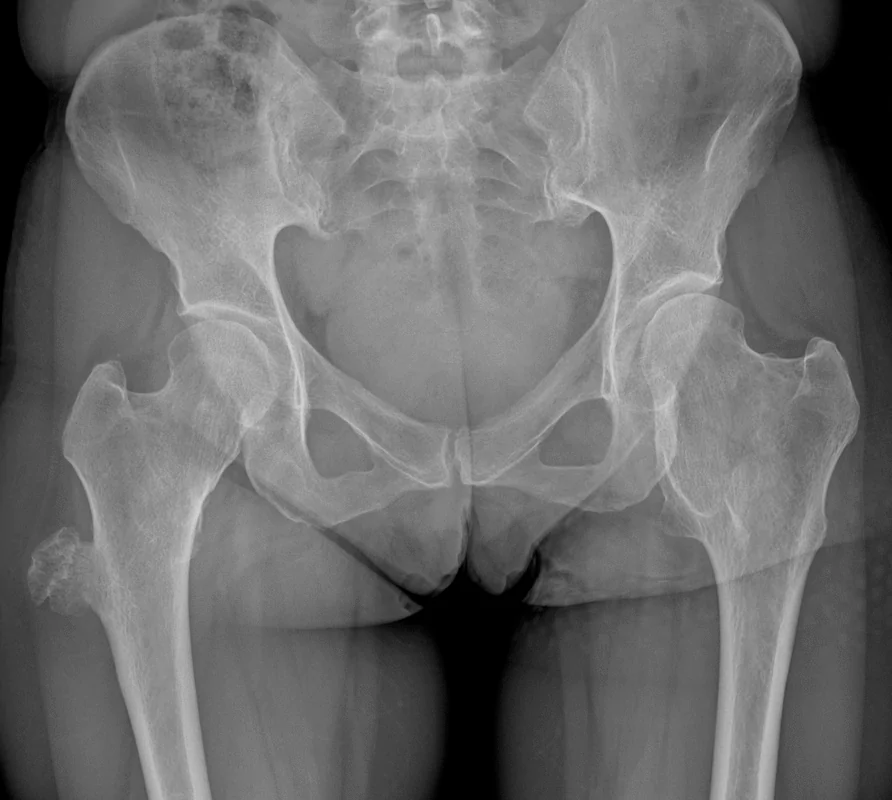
Anterior–posterior radiograph of the pelvis at admission. A large osteochondroma of the femoral neck at the calcar region causes the femoroacetabular impingement affecting the patient
